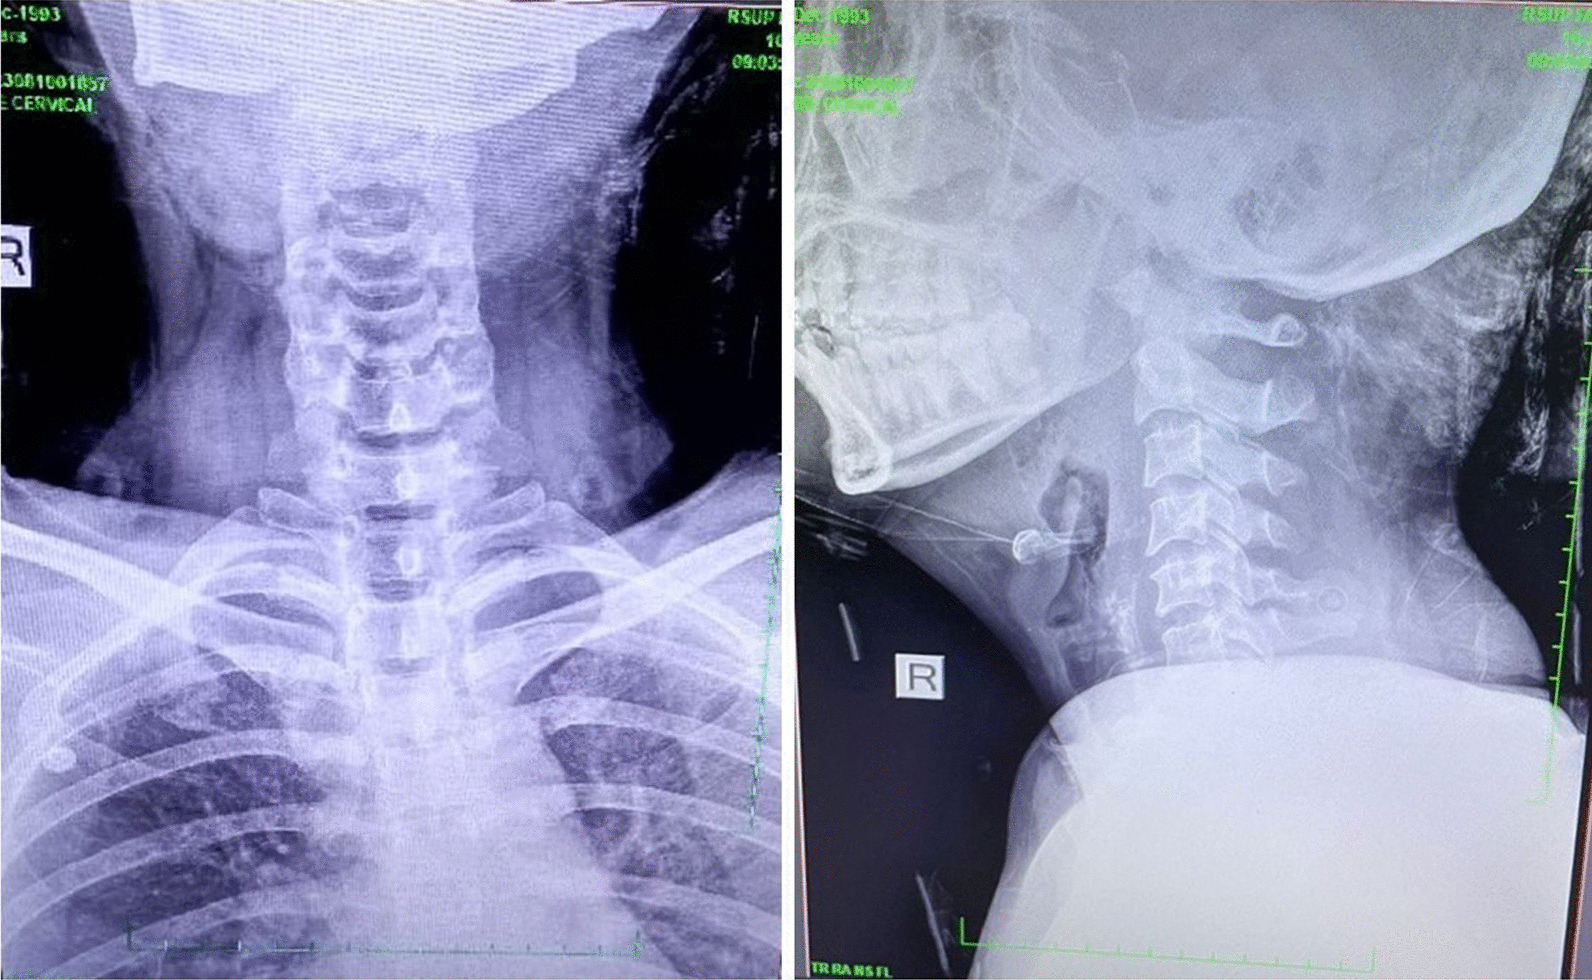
Cervical X-ray showed grade 1 C2–C3 anterolysthesis with prevertebra soft tissue swelling, suspected hematoma
